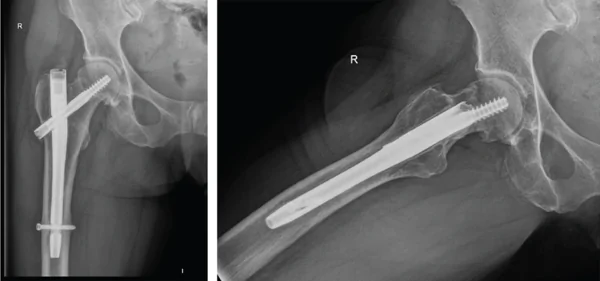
AP and lateral radiographs at 6 weeks following subsequent injury.
